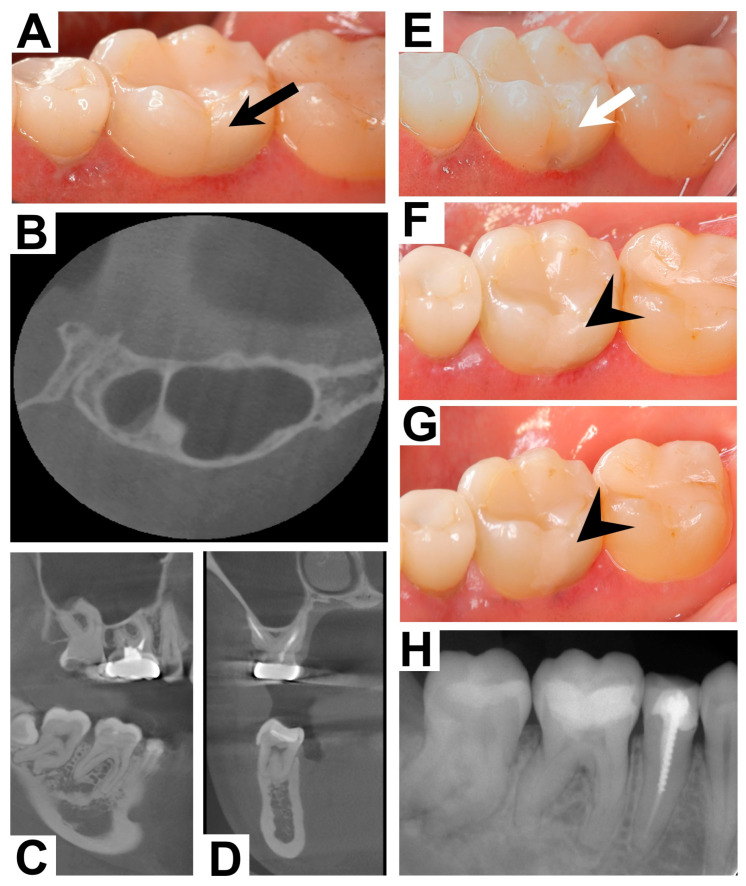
(A) An intraoral photograph of the crack line in the lingual surface of the right mandibular first molar (black arrow); (B–D) The cone-beam computed tomography (CBCT) images of the right mandibular first molar and right maxillary sinus; (E) Following the administration of local anaesthesia, a diamond bur was used to prepare a shallow groove along the crack line to facilitate adhesion (white arrow). (F) After appropriate acid etching, a methyl methacrylate (MMA)-based dental adhesive material (Super-bond; Sun Medical Co., Ltd., Shiga, Japan) was used to fill the groove (arrow head); (G,H) Patient’s prognosis monitored regularly (arrow head), demonstrating favourable progression and absence of pain recurrence (3 years later).
